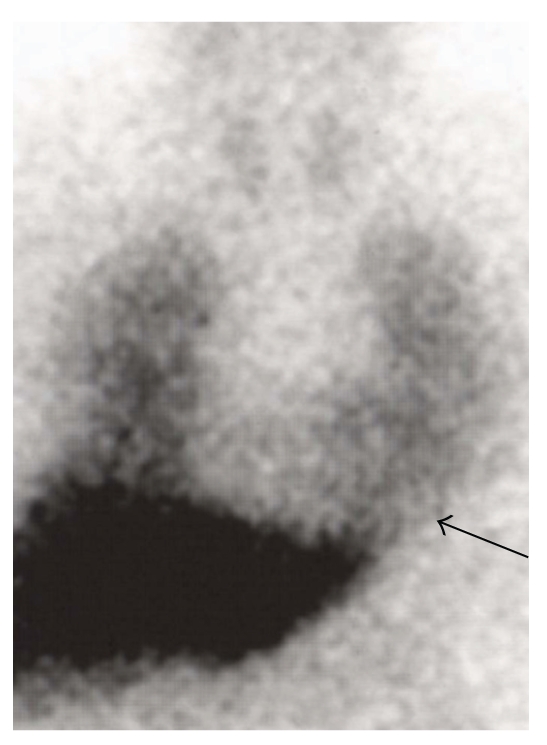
MIBG scintigraphy in tremor dominant PD. MIBG scintigraphy in a 62-year-old male patient with tremor dominant PD, Hoehn and Yahr stage I. The myocardial MIBG uptake (black arrow) is well visible but pathologically reduced.
